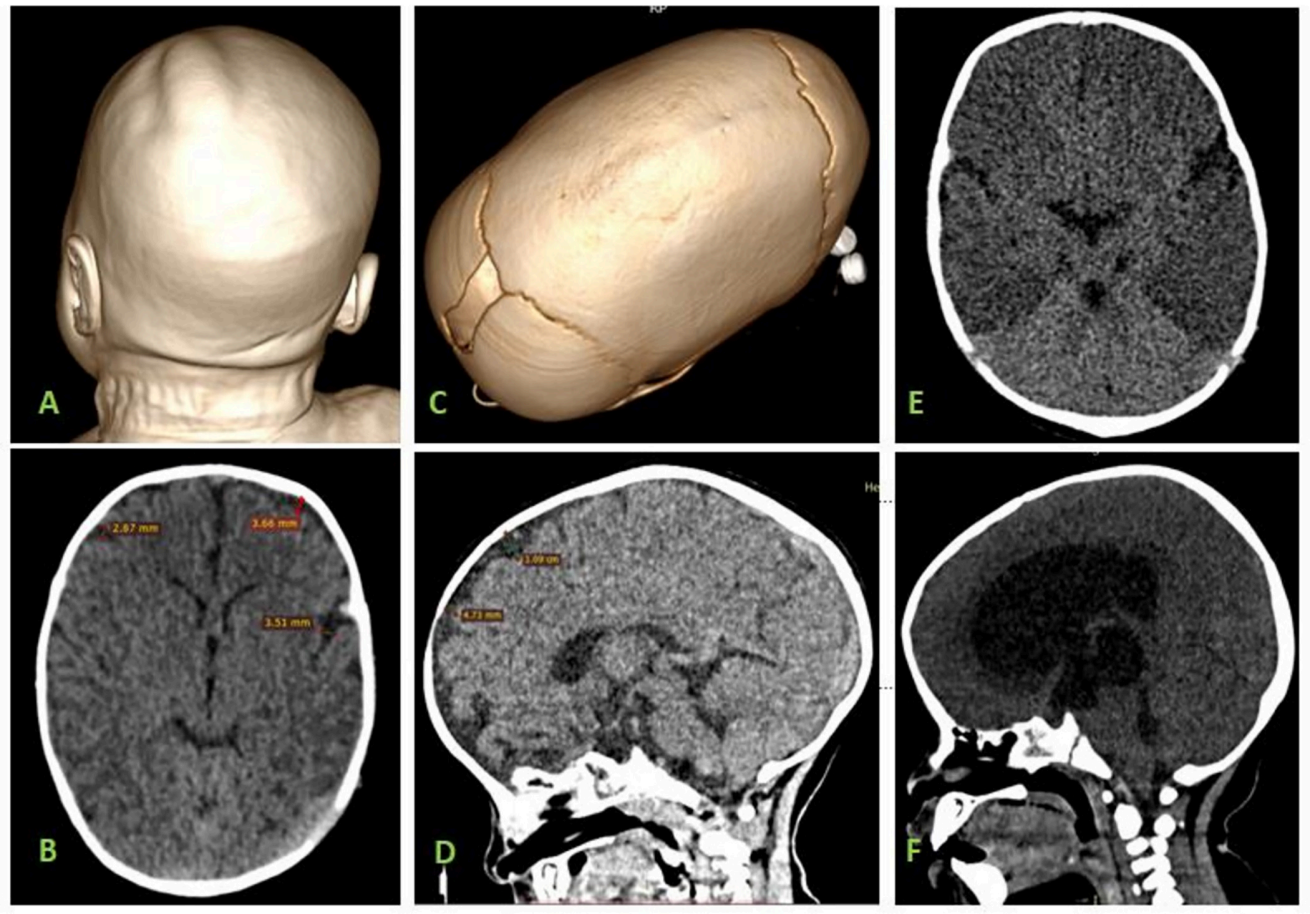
Selected head CT scans showing varying causes of brain volume loss. A: Volume rendering 3D CT scan image of a 3 months’ child with birth related head injury presenting with cephalohematoma at the vertex. B: Axial CT scan image of the same child A showing diffuse reduction in brain volume in cortical regions as evidenced by prominent sulci. C: Volume rendering 3D image of 1-year male child showing premature closure of the sagittal suture with overall increase in anterior-posterior diameter of the head(craniosynostosis). D: Sagittal CT brain image of the same child C showing loss of brain volume in the frontal lobes showing gross deviation from the calvarium with prominent CSF spaces. E: A 2 months’ female child presenting with diffuse reduction in cerebral hemispheric density sparing the cerebellum (White cerebella sign) representing hypoxic ischemic encephalopathy with early bi-temporal cerebral atrophy. F: A 4 months’ male child showing increased intracranial pressure evidenced by effacement of sulci and gyri as a result of ballooning of the lateral and 3^rd^ ventricles. The 4^th^ ventricle is small due to obstructive hydrocephalus following aquiductal stenosis.
